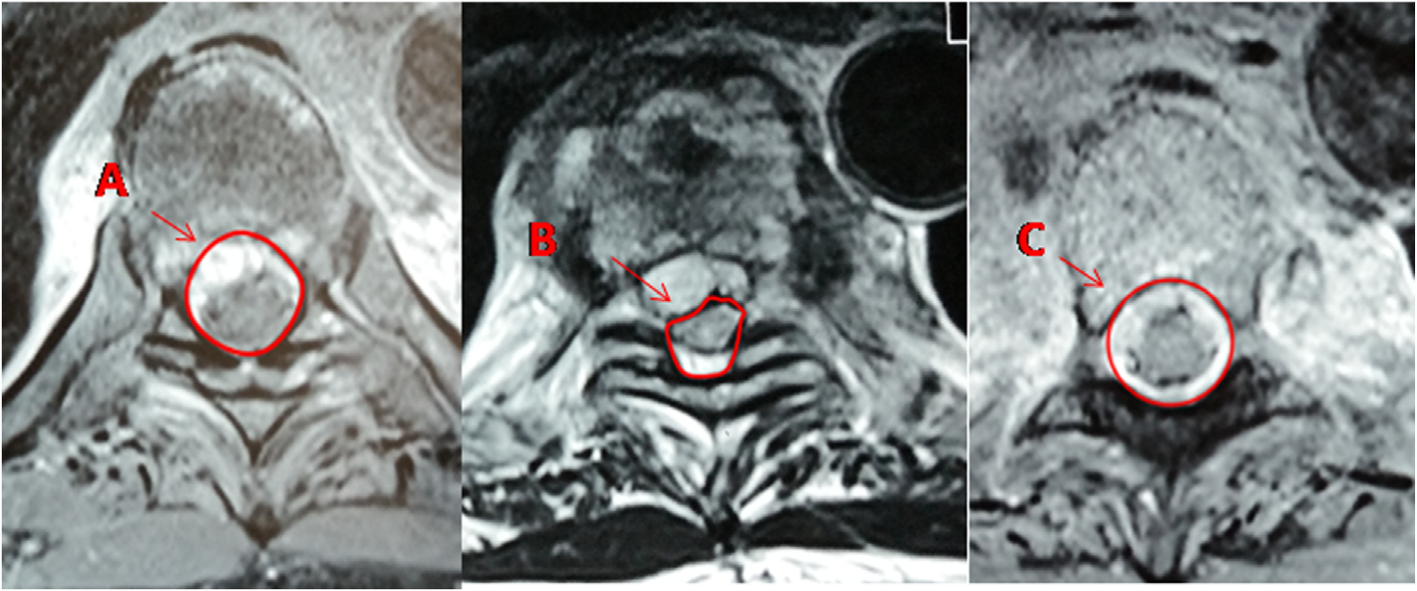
Measurement of canal encroachment in axial section of MRI. *A* = cross-sectional area of canal one level above the site of maximum compression, *B* = area occupied by spinal cord at maximum compression, *C* = cross-sectional area of canal one level below site of maximum compression. Average canal area (*D*) = *A* + *C*/2. Percentage of canal encroachment = *D* − *B*/*D* × 100.
